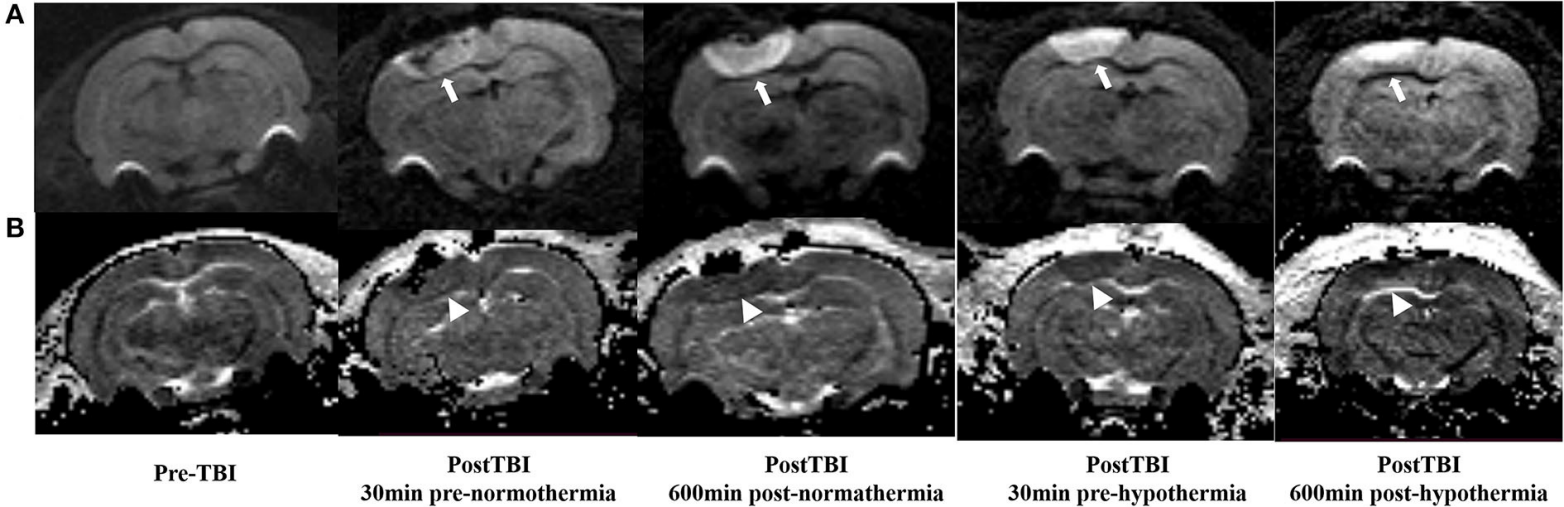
DWI and calculated ADC maps acquired before and 30 min, 10 h post-TBI. Edematous tissue of traumatic lesion appears hyperintense in diffusion-weighted MRI [(A), arrows] and shows hypointense in ADC maps [(B), arrowheads].
